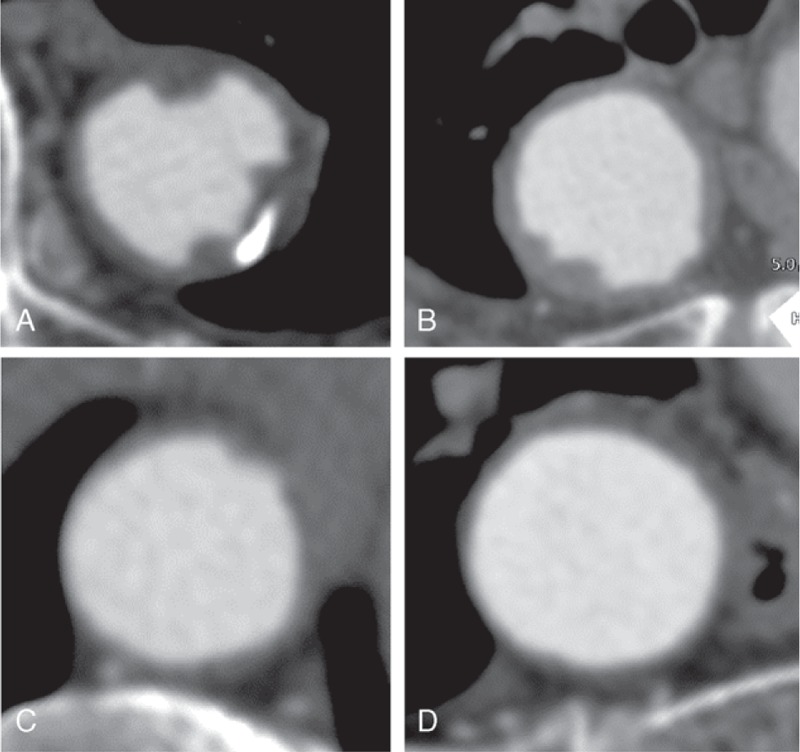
Examples of multiplanar reconstruction computed tomography images perpendicular to the aortic centerline. (A) A patient with both hypertension and coronary artery disease (CAD) (shagginess score, 12.434). (B) A patient with CAD and without hypertension (shagginess score, 8.538). (C) A patient with hypertension and without CAD (shagginess score, 8.133). (D) A patient without hypertension or CAD (shagginess score, 7.705).
